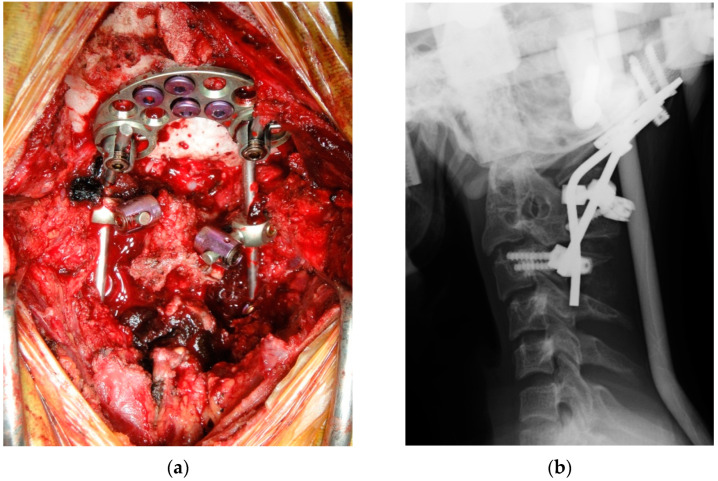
(a) Intraoperative findings of posterior occipitocervical fixation; (b) postoperative lateral X-ray.
